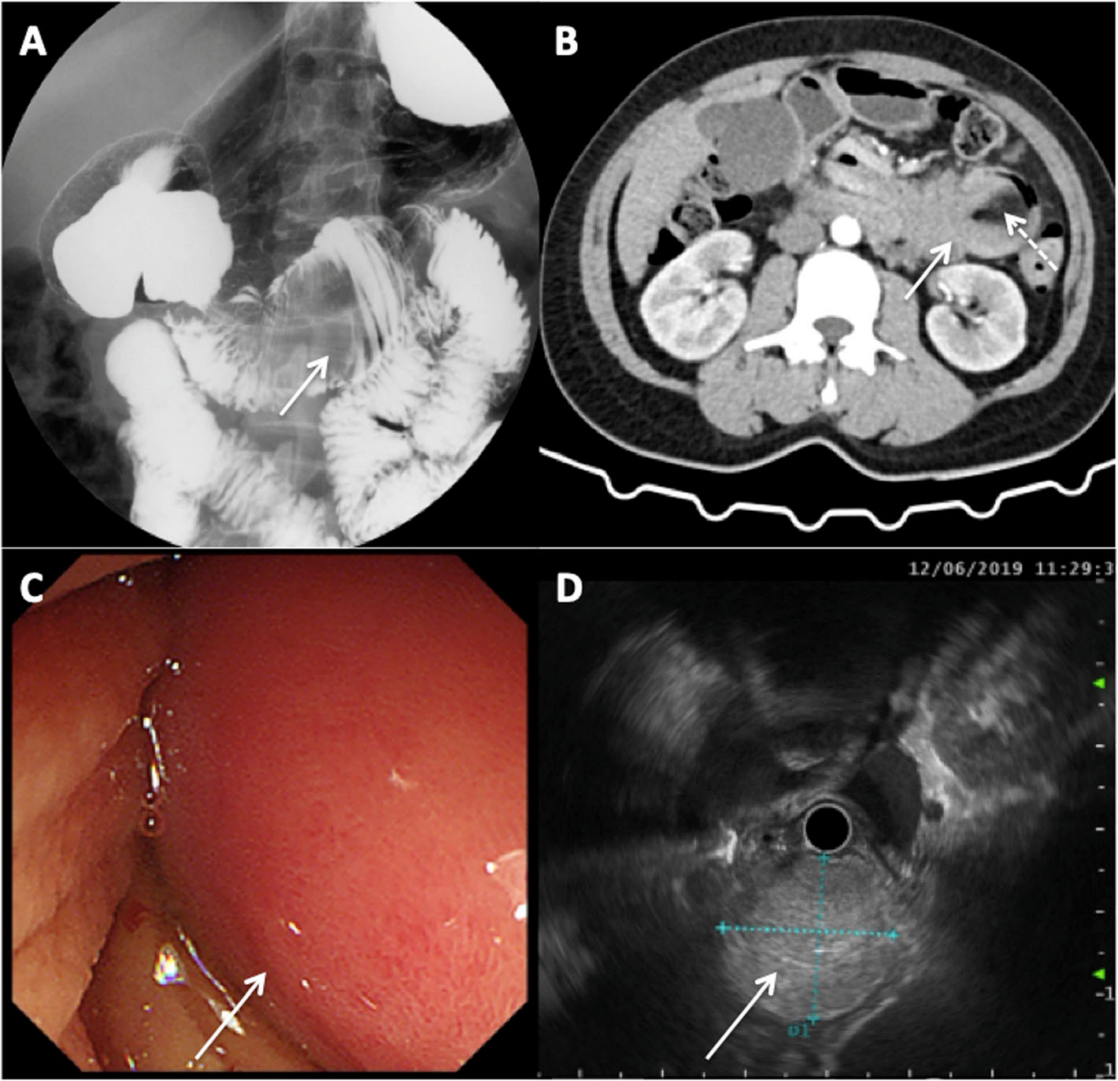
Endoscopy and radiography examination. a Upper gastrointestinal tract showed filling defect (arrow) in the horizontal and ascending part of duodenum; b Abdominal enhanced CT showed a cuff-like change (solid arrow) of intestinal segments in the left upper abdomen with fat-density opacity (dashed arrow) seen in the lumen; c Duodenoscopy showed “a large mass” (arrow) in descending part; d Ultrasound endoscopy showed a medium-high echo mass (arrow) derived from the submucosa)
